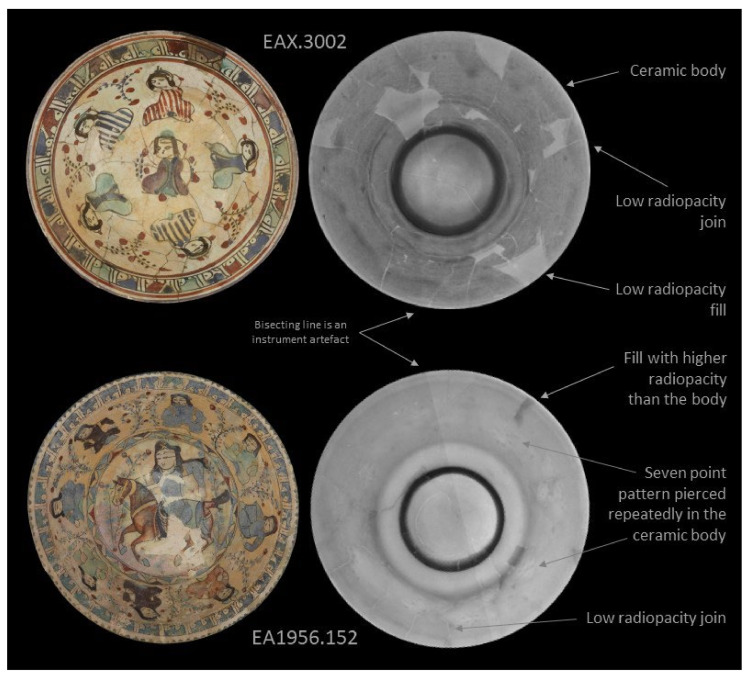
Results of digital photography on the left compared to digital radiographs on the right for EAX.3002 and EA1956.152.
